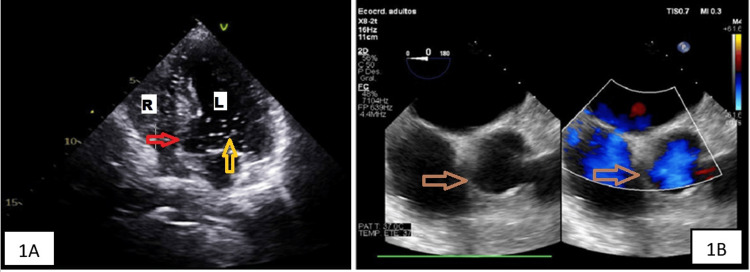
1A: Bubble echocardiogram demonstrating early and massive passage of bubbles from right to left cavities, suggesting intracavitary shunt compatible with the diagnosis of PFO in patient 1. 1B: PFO visible through transesophageal echocardiography R: right cavities, L: left cavities, PFO: patent foramen ovale, red arrow: PFO, yellow arrow: bubbles in left cavities, brown arrow: PFO visible through transesophageal echocardiography
